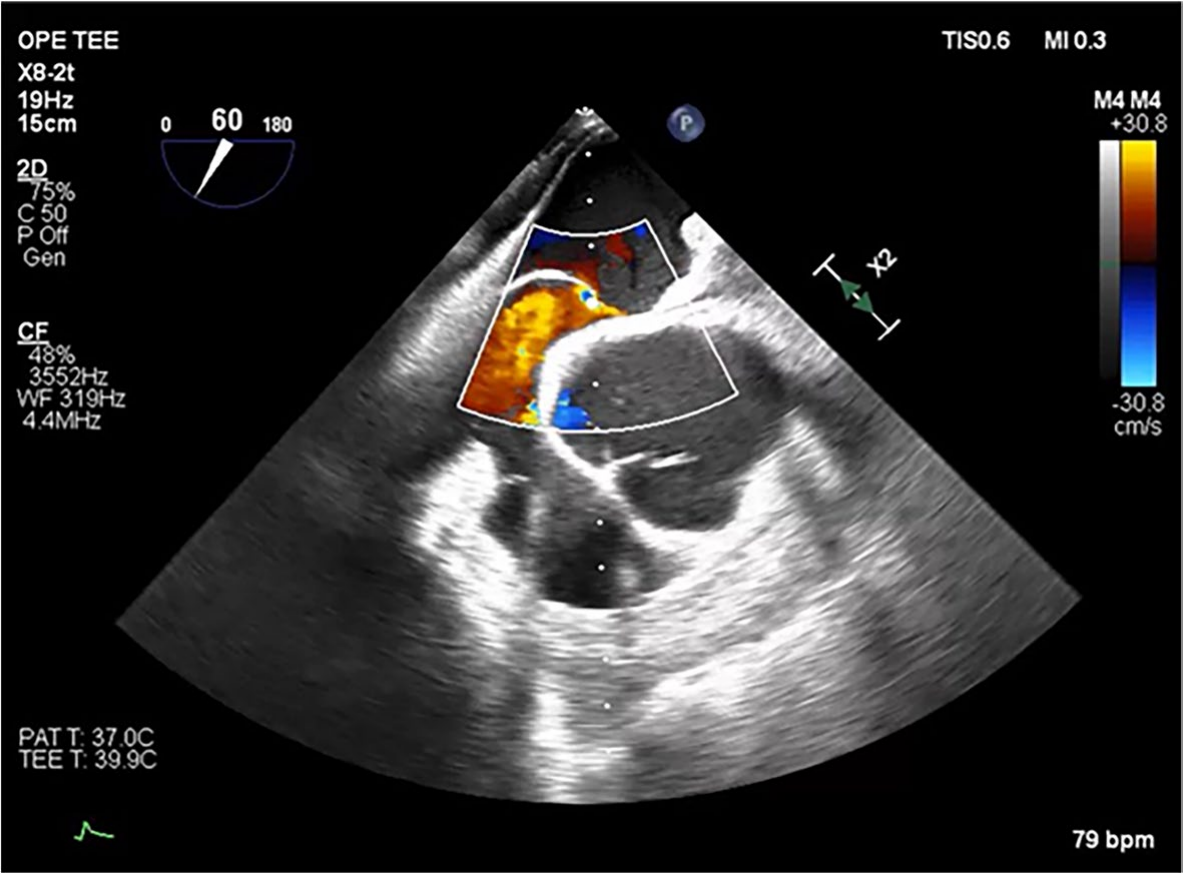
Doppler image of aortic valve short axis view on transesophageal echocardiography. A marked right-to-left shunt through the patent foramen ovale
